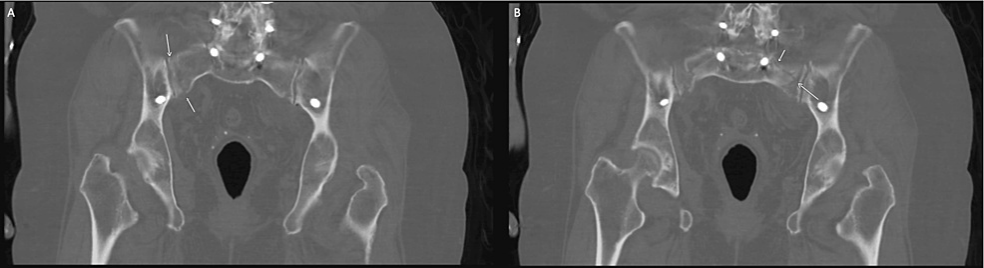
Coronal reformat of the CT scan of the pelvis performed on the day of the fall. (A) Right-sided fracture of the sacral ala and (B) left-sided fracture of the sacral ala with extension to the sacroiliac joint are demonstrated (white arrows).
